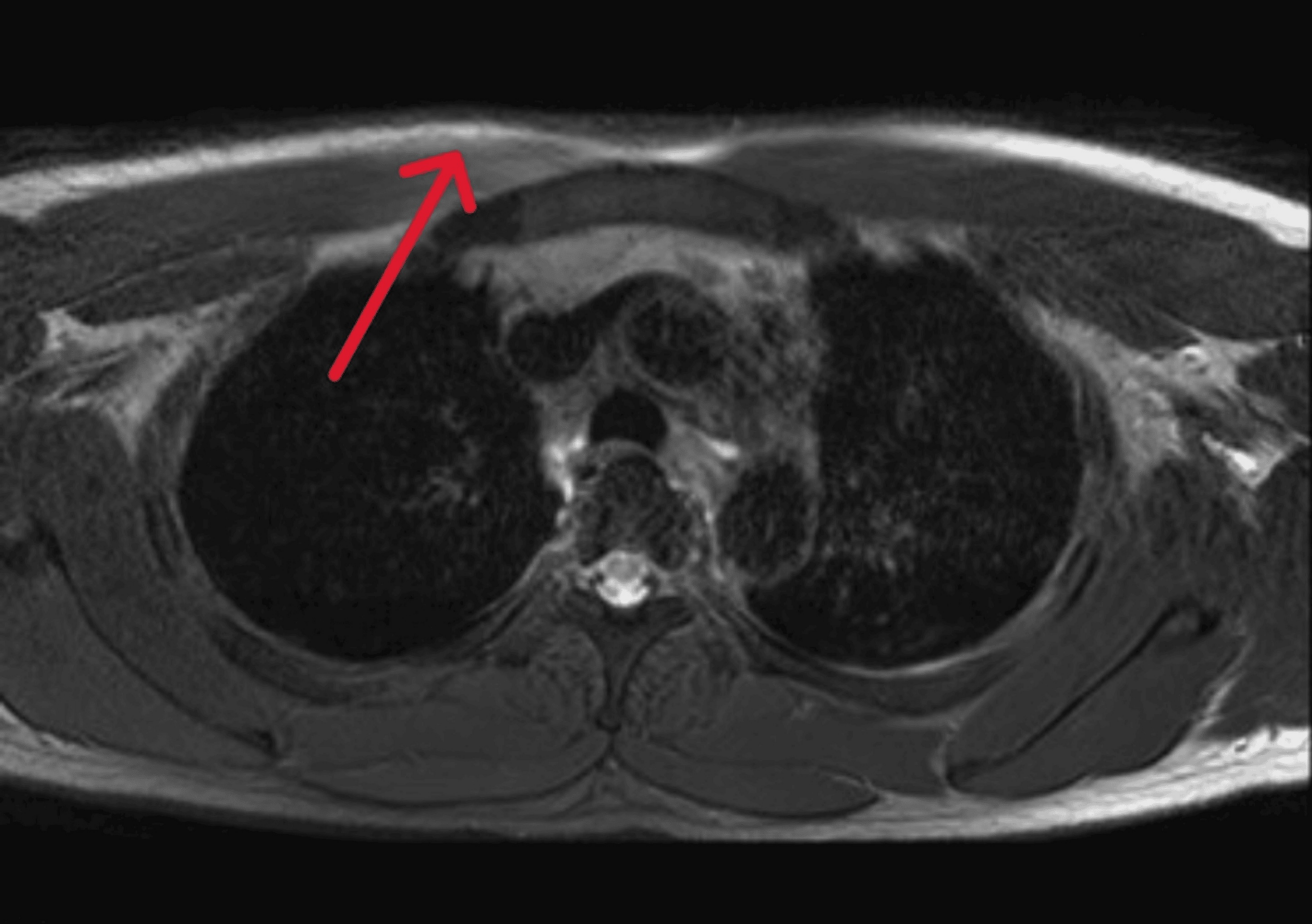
Axial short tau inversion recovery (STIR) MRI revealed deep soft tissue edema in the right proximal pectoralis major muscle (red arrow).
